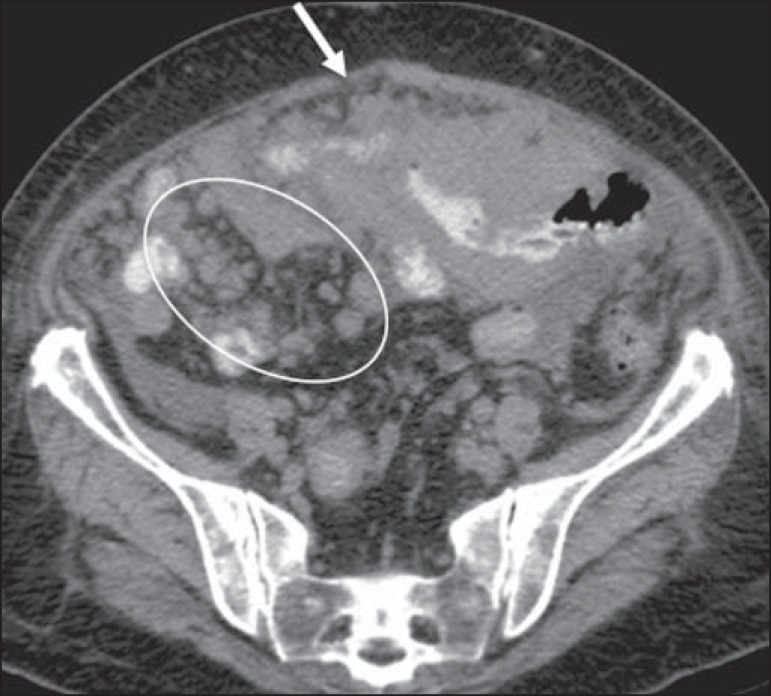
Renal lymphoma. Contrast-enhanced CT of the abdomen, in the axial plane
